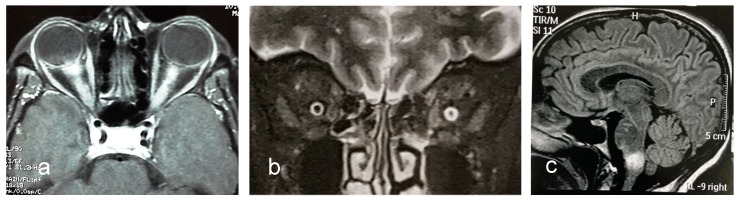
Examples of MRI abnormalities in anti-MOG syndrome. (a). Axial T1-weighted MRI reveals longitudinal extensive gadolinium enhancement of both optic nerves. (b). Coronal T2-weighted MRI shows hyperintense thickening of perioptic nerve sheath. (c). Sagittal T2/FLAIR-weighted image shows large fluffy lesion in the medulla.
